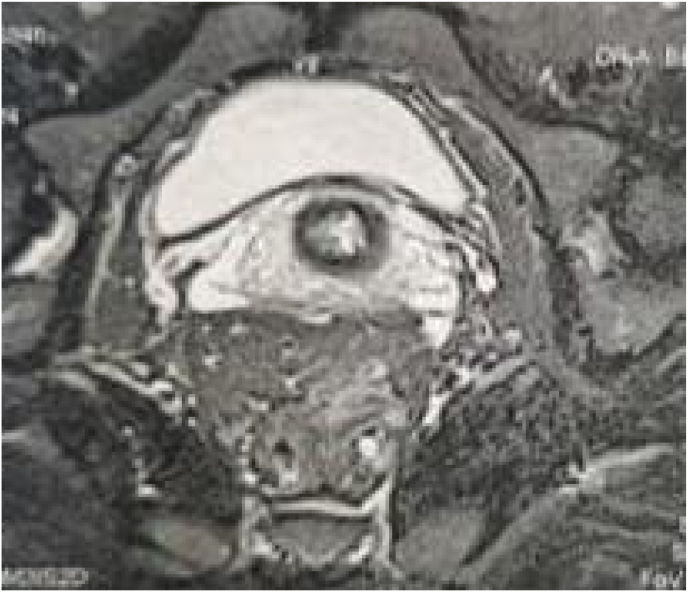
MRI of a patient showing a lower rectal tumor located 4 cm from the anal margin classified as T3N2Mx.
